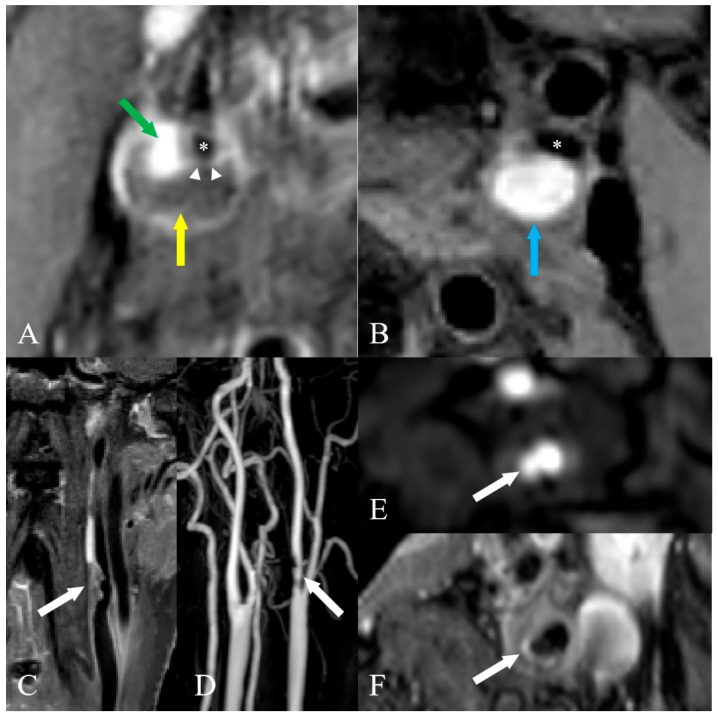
Representative examples of vulnerable features of carotid plaques. (A). CE-T1WI demonstrates severe stenosis at the origin of the right internal carotid artery (white *). Focal inflammation (green arrow) within the LRNC (yellow arrow). The FC (white short arrow) shows uneven thickness. (B). SNAP reveals severe stenosis at the origin of the left internal carotid artery (white *). A hyperintense area suggests extensive IPH (blue arrow). (C–F). Severe stenosis at the origin of the left internal carotid artery with FC discontinuity (ruptured FC, ulceration). CE-MRA reveals ulcerated stenosis (white arrow, (C)). Coronal VISTA (white arrow, (D)), axial 3D TOF (white arrow, (E)), and axial CE-T1WI (white arrow, (F)) demonstrate plaque surface ulceration. CE-T1WI, contrast-enhanced T1-weighted imaging; SNAP, simultaneous non-contrast angiography and intraplaque hemorrhage; 3D, three-dimensional; TOF, time of flight; VISTA, volume isotropic turbo spin echo acquisition; LRNC, lipid-rich necrotic core; IPH, intraplaque hemorrhage; FC, fibrous cap.
